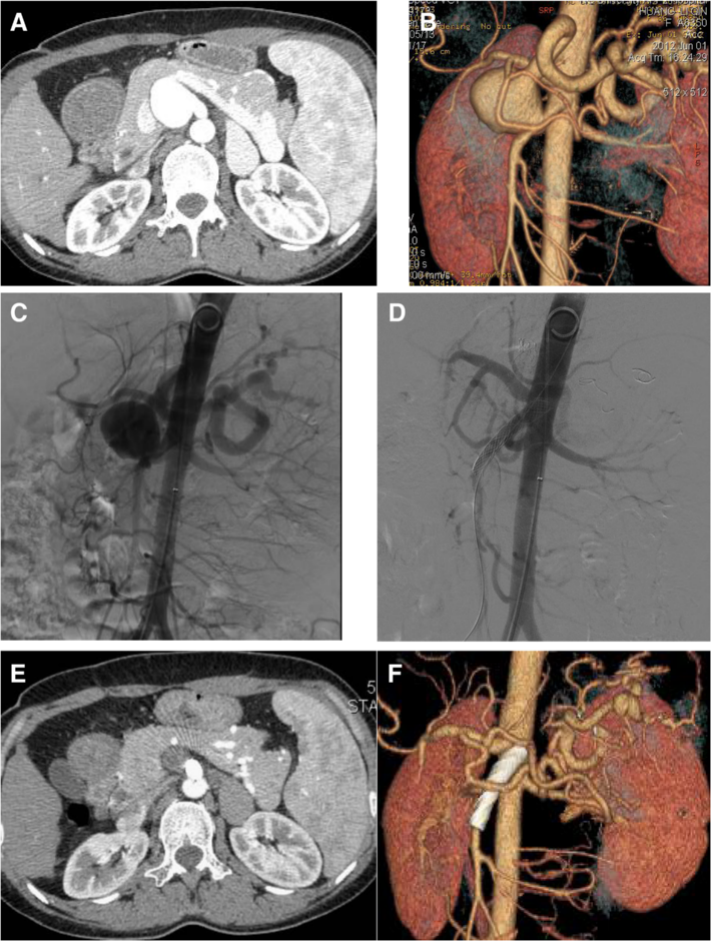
The image finding of case two during preoperation, intraoperation and follow-up. A, B and C, Preoperative CTA and intraoperative DSA demonstrated a 36 mm × 32 mm SAA near the origin of the SMA and the proximal caliber of the SAA was 11 mm. D, The completion aortogram revealed no evidence of endoleak; disappearance of the aberrant SAA lumen and patency of the SMA and the branches immediately after the stent deployment. E and F, Follow-up abdominal CT scan in 12 months postoperatively confirmed an occluded aneurysm sac with marked shrinkage and patency of the SMA.
